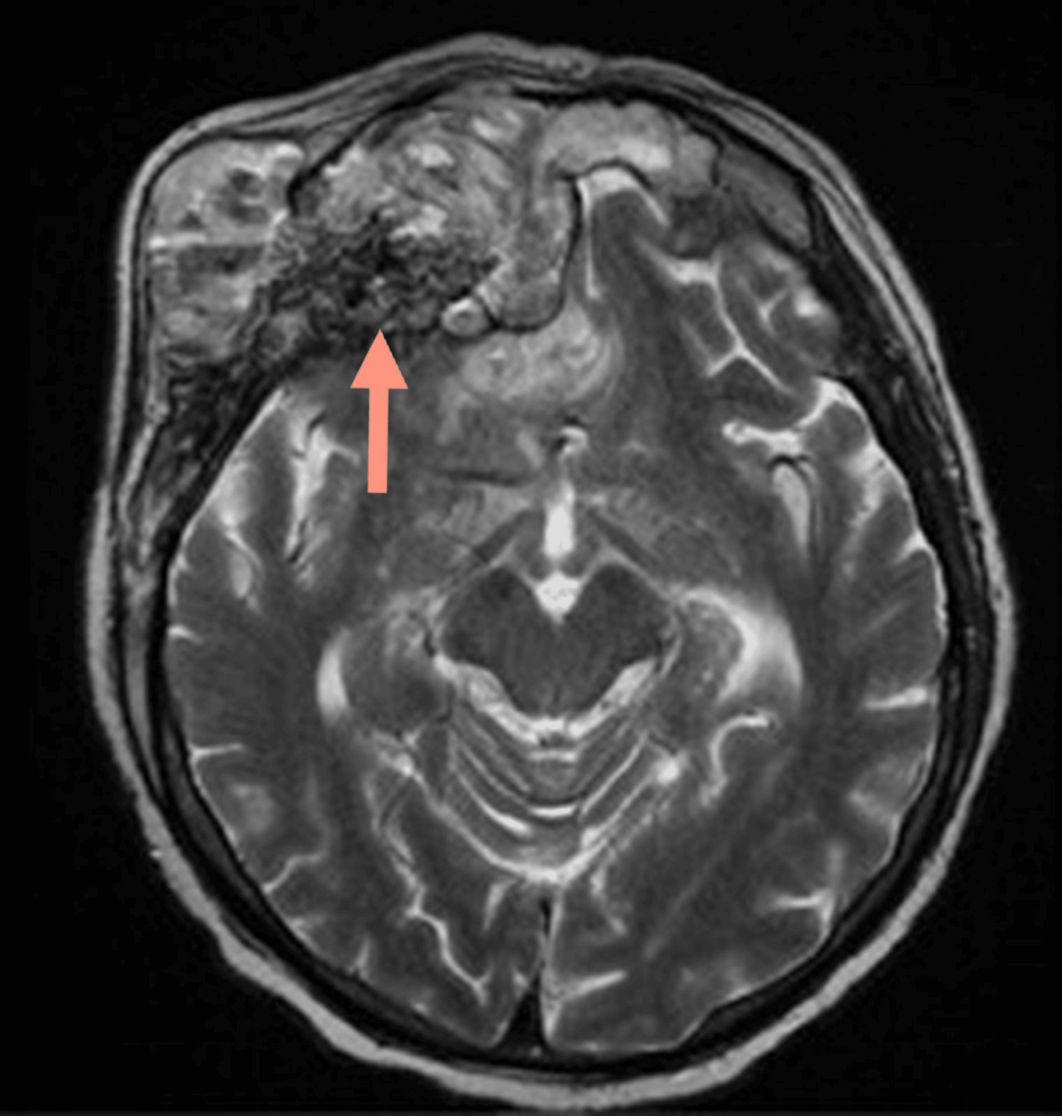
Axial T2-weighted imaging showing dark signal within the mass (red arrow).
